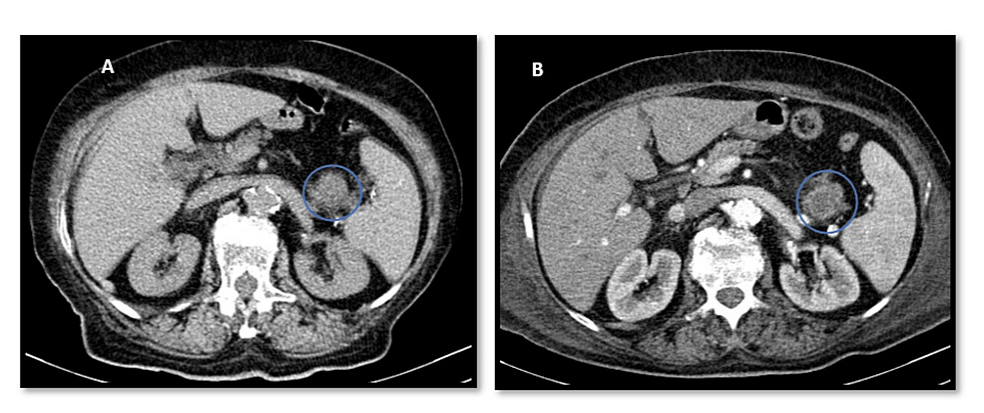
Pancreatic lesion in the first (A) and last (B) computed tomography scans. (B) Lesion is more heterogeneous and has some contrast enhancement.
